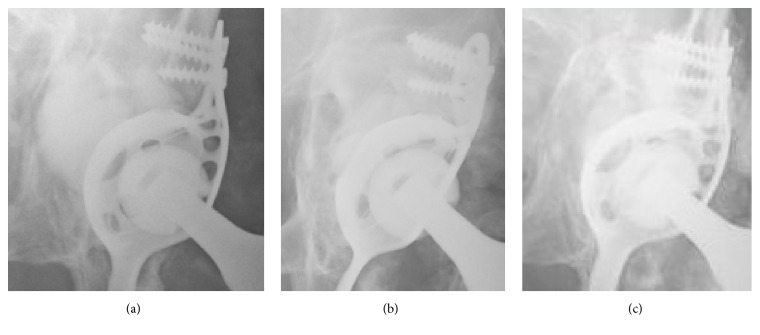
Enlargement of the a.p. X-rays of the left hip: at (a) two days, (b) one year, and (c) two years and eight months postoperatively: continuing remodelling of CERAMENT|BONE VOID FILLER into bone.
